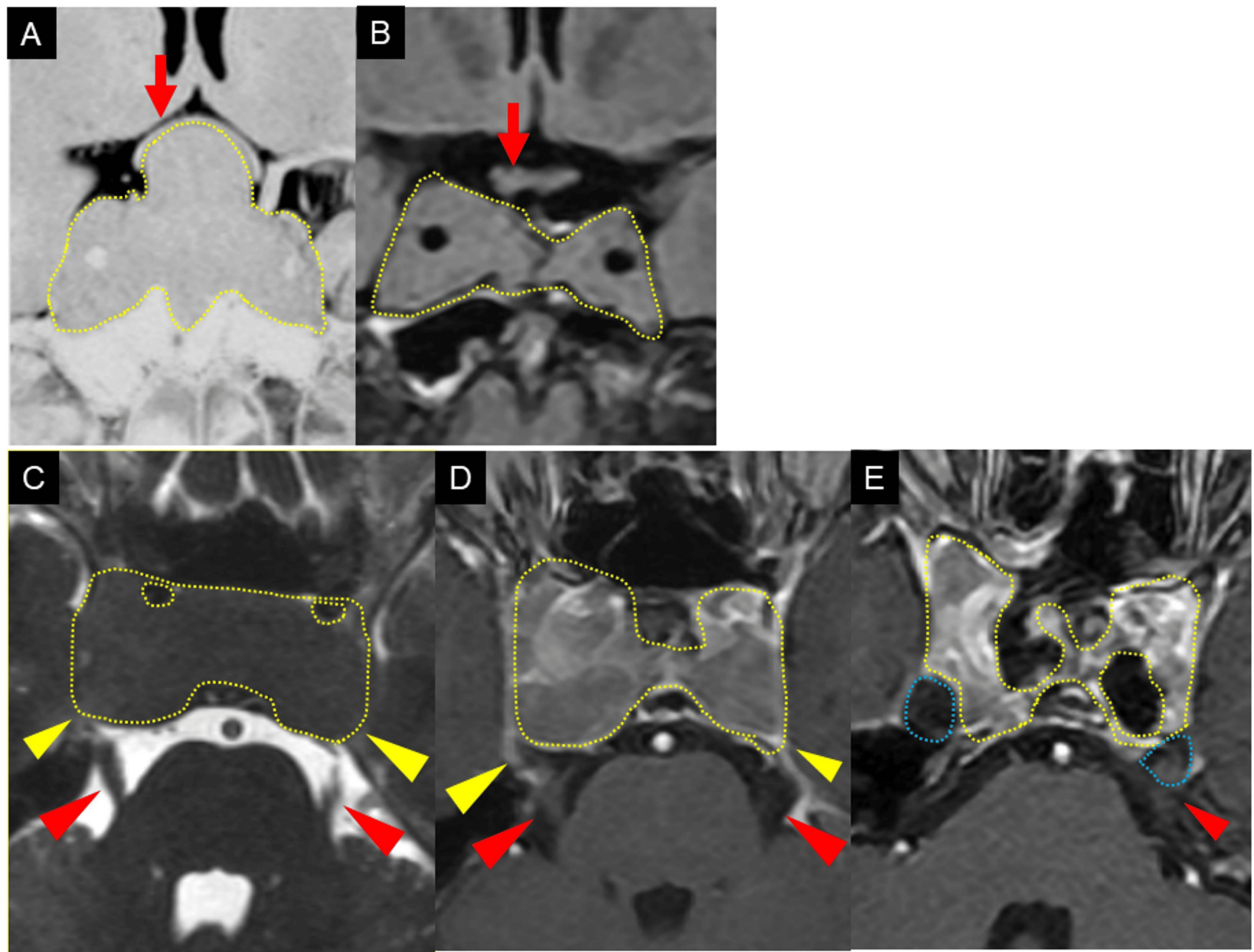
Magnetic resonance imaging (MRI) before surgery, after the first surgery, and after the second surgery Yellow dashed line: pituitary tumor; blue dashed line: Meckel's cave; red arrow: optic chiasm; red arrowhead: trigeminal nerve; yellow arrowhead: bone/dura (A) Preoperative coronal view showing strong optic chiasm compression and deformation by the pituitary tumor. At that time, no symptoms were present. (B) Postoperative coronal view (first surgery) showing resolved optic chiasm compression. Postoperatively, the patient developed photophobia, ocular pain, and difficulty opening the eyelids. (C) Preoperative axial view showing Meckel's cave obliteration by the pituitary tumor and presumed trigeminal nerve compression. At that time, no symptoms were present. (D) Postoperative axial view (first surgery) showing Meckel's cave obliteration and still presumed trigeminal nerve compression. Postoperatively, the patient developed photophobia, ocular pain, and difficulty opening the eyelids. (E) Postoperative axial view (second surgery) showing restored Meckel's cave and relieved trigeminal nerve compression. Following the second surgery, photophobia, ocular pain, and difficulty opening the eyelids resolved completely.
